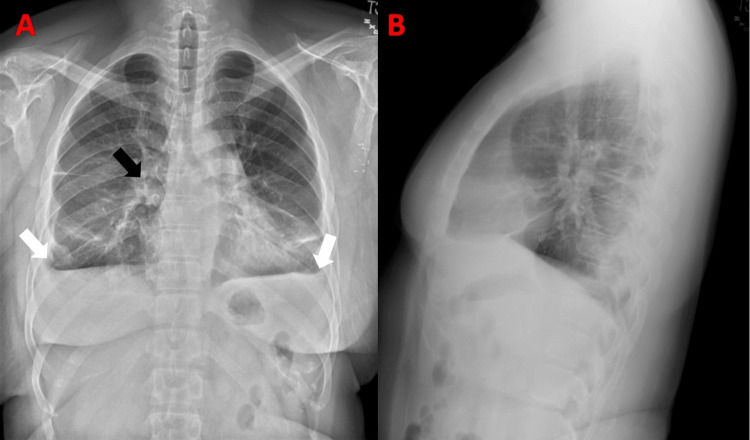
Chest x-ray posteroanterior (A) and lateral (B) views demonstrating patchy opacities over the hilar region (black arrow), haziness and blunting of costophrenic angle (white arrows) suggestive of pleural effusion. Possible cardiomegaly.
